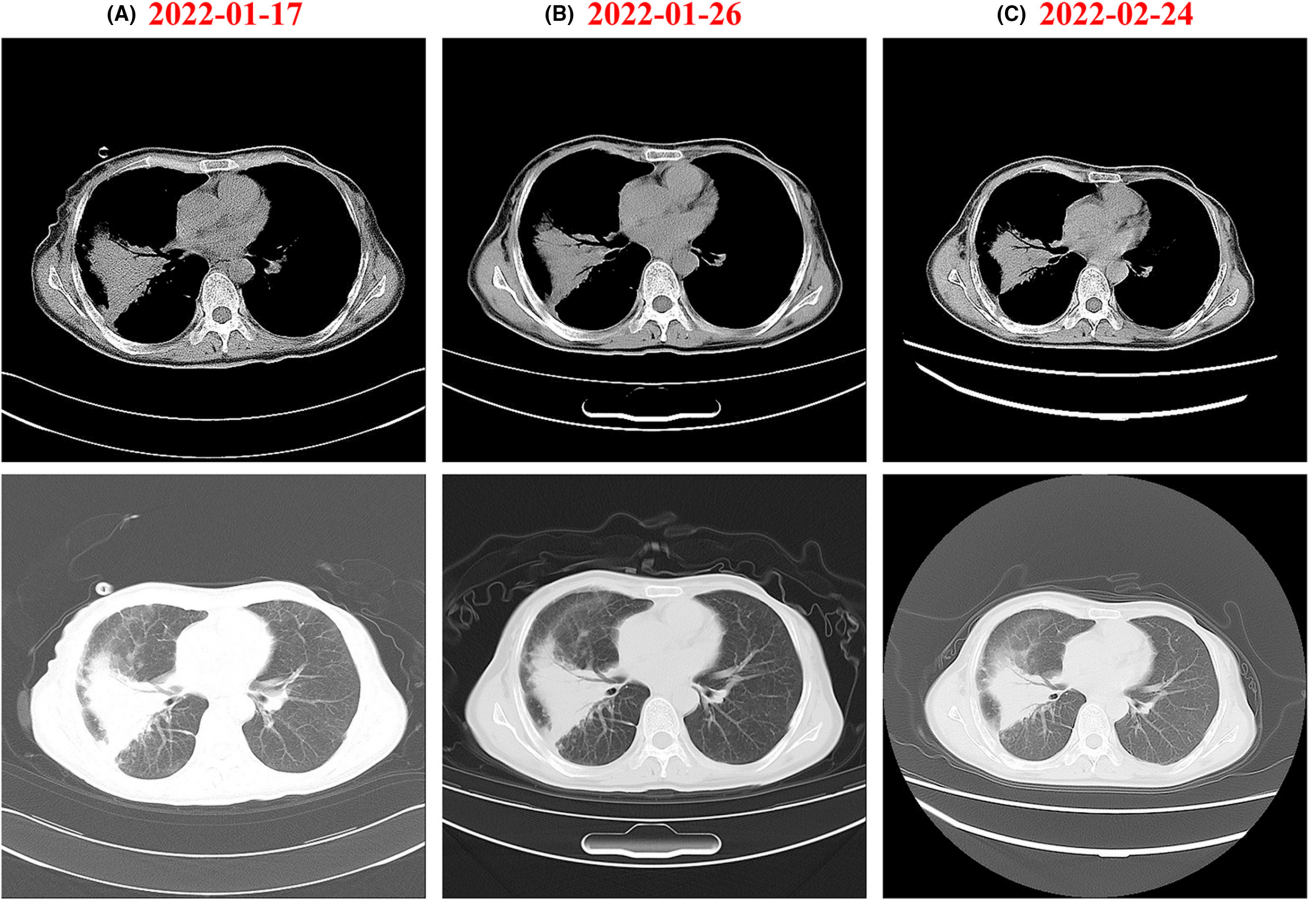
Chest CT scans before and after anti‐infective drug therapy. (A) CT scan performed on January 17, 2022, before treatment. (B) Follow‐up CT scan performed on January 26, 2022, after treatment. (C) Follow‐up CT scan performed on February 24, 2022.
